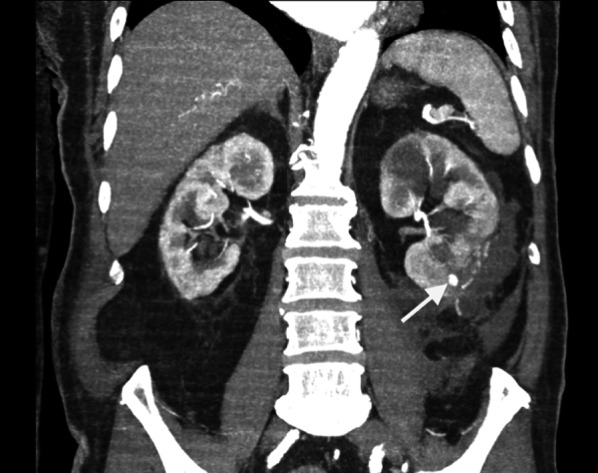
Arterial phase CT image of a pseudoaneurysm (white arrow) post-laparoscopic partial nephrectomy of the lower pole of the left kidney
